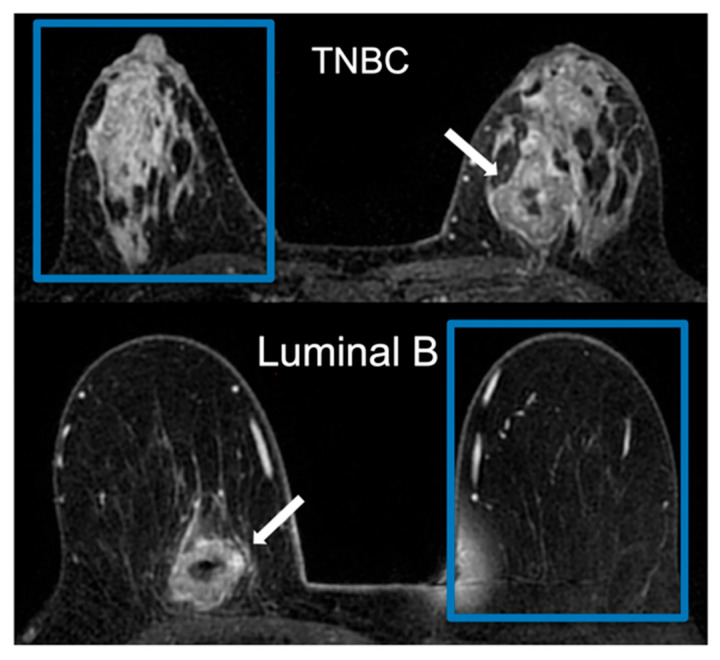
Example of a post-contrast axial T1-weighted image depicting an irregular lesion in the mid-inner quadrant of the left breast, which was correctly predicted by our radiomic machine-learning model as triple-negative breast cancer (TNBC), and an example of a post-contrast axial T1-weighted image depicting an irregular lesion in the lower-inner quadrant of the right, which was correctly predicted by our radiomic machine-learning model as luminal B cancer (ER-positive, PR-negative, and HER2-positive breast cancer). The fibroglandular tissue of the contralateral breast was segmented as in Figure 1.
